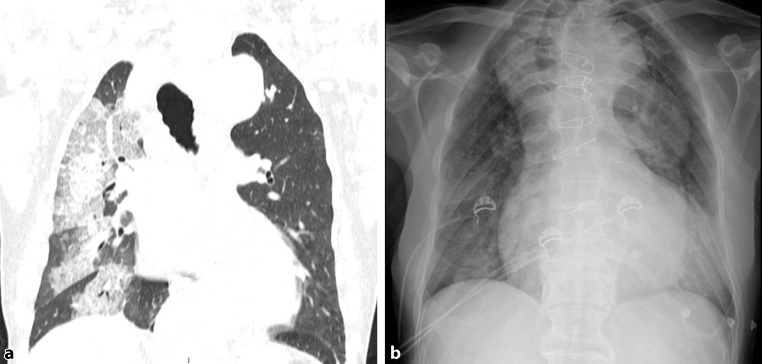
a Thoracic CT scan showing right pulmonary consolidations as a sign of aspiration pneumonia. b Chest X‑ray showing resolution of pneumonia
